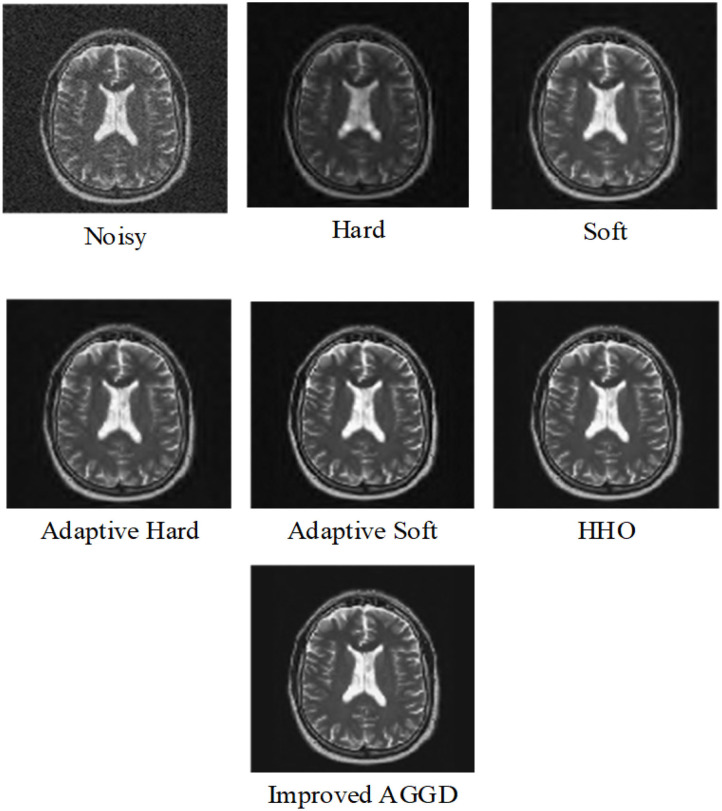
Comparison of visual inspection between different noise reduction methods for MRI Brain Image 3 for variance 0.03.
